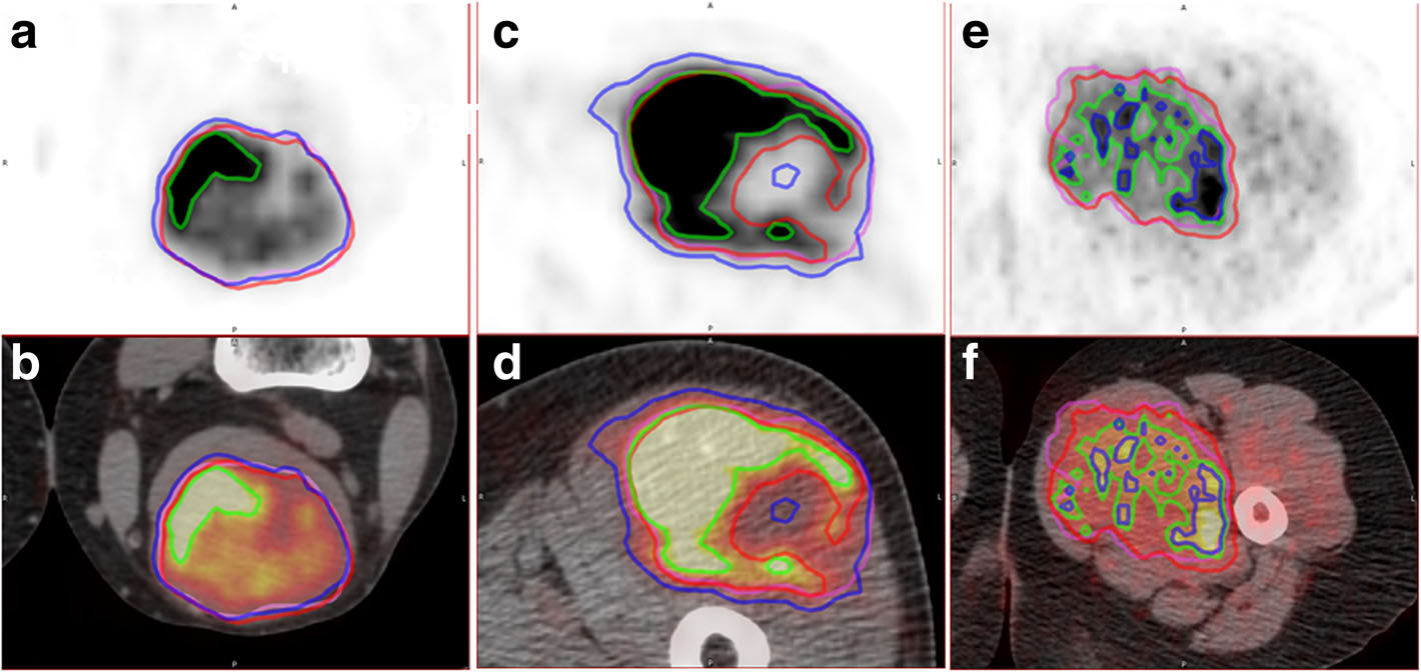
Images of representative cases (a, c, and e axial ^18^F-FDG PET image; b, d, and f axial fusion image). a, b A 54-year-old male with synovival sarcoma in the left popliteal fossa (SUV_max_ 28.71). The ratios of MTV to GTV for MO-PET, SUV 2.0, 30% SUV_max_, and PET Edge™ are 1.05, 1.06, 0.25, and 0.97, respectively. c, d A 64-year-old male with extraskeletal osteosarcoma in the right thigh (SUV_max_ 27.77). The ratios of MTV to GTV for MO-PET, SUV 2.0, 30% SUV_max_, and PET Edge™ are 0.90, 1.62, 0.57, and 1.17, respectively. e, f A 42-year-old female with pleomorphic leiomyosarcoma in the left thigh (SUV_max_ 5.47). The ratios of MTV to GTV for MO-PET, SUV 2.0, 30% SUV_max_, and PET Edge™ are 1.13, 0.24, 0.46, and 0.99, respectively. Red line, MO-PET; blue line, SUV 2.0; green line, 30% SUV_max_; pink line, PET Edge™
